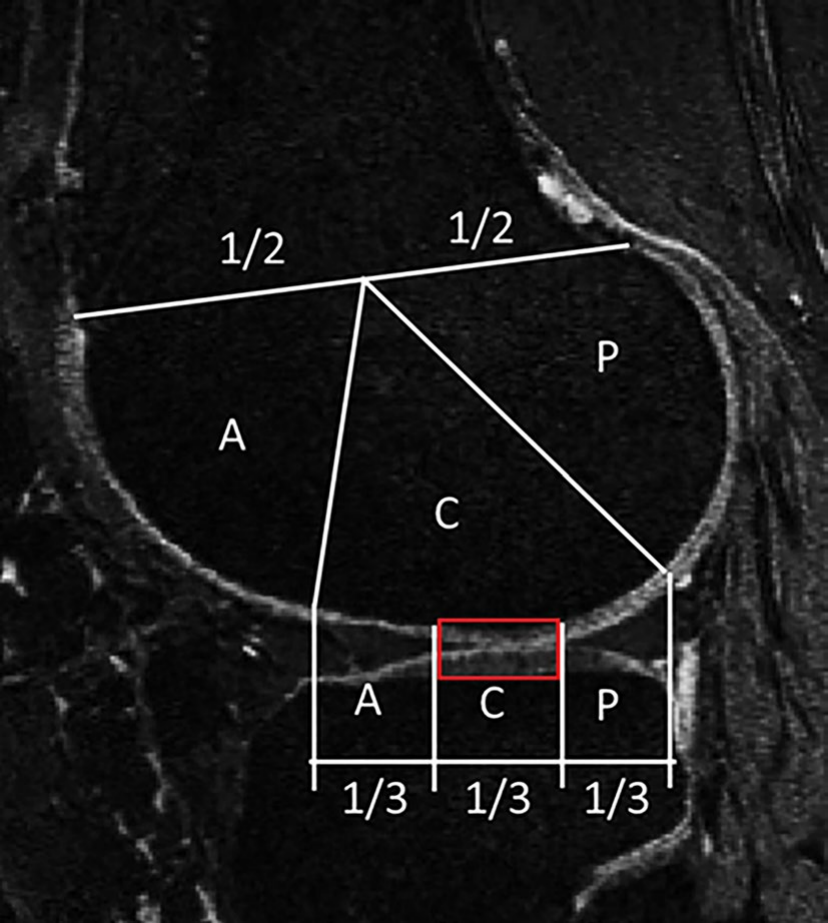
MRI sagittal showing in red the region of interest used to define the volumetric measure of cartilage degeneration. *A* anterior, *C* central, *P* posterior
